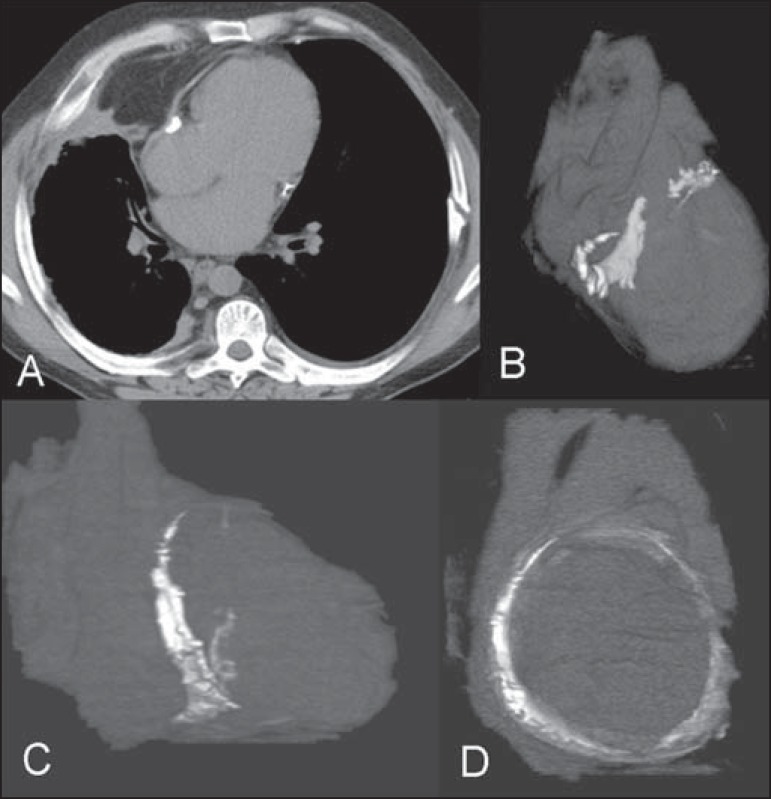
Axial computed tomography image (A) and volume-rendered
